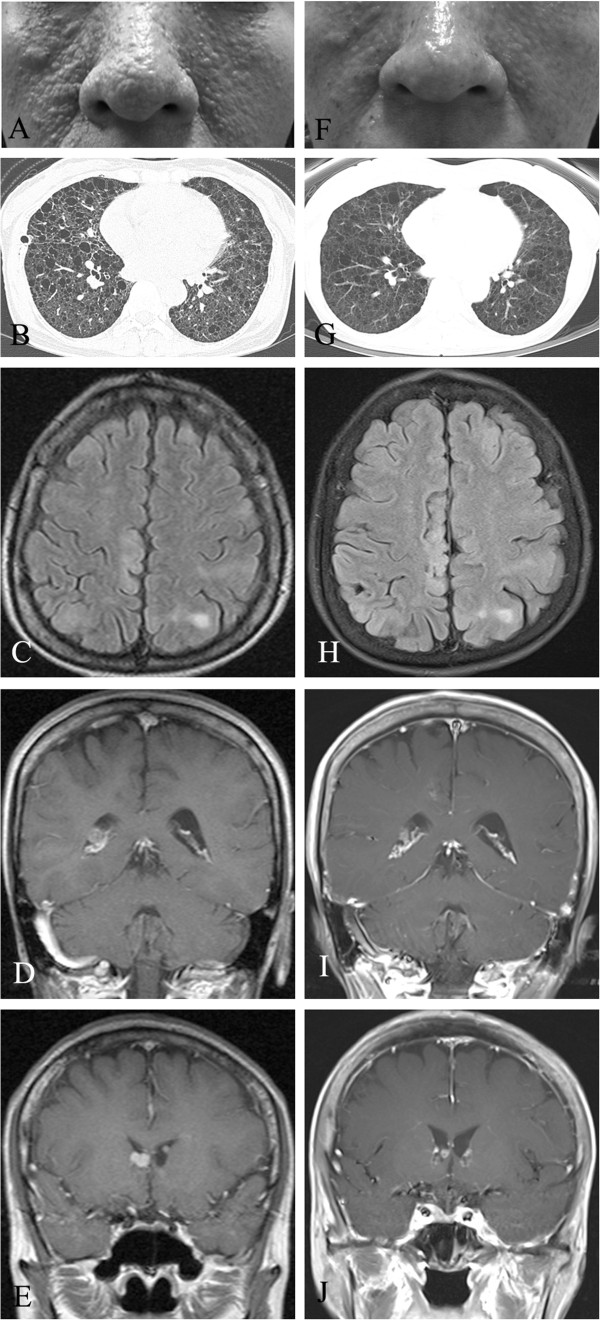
Clinical features of tuberous sclerosis complex at diagnosis and after treatment with everolimus. (A) Photograph of the patient’s facial fibroadenoma. (B) Chest computed tomographic scan of multiple cystic lesions consistent with lymphangioleiomyomatosis. (C) through (J) Brain magnetic resonance imaging scans of the patient. (C) Cortical tubers. (D) Multiple subependymal nodules. (E) Subependymal giant cell astrocytoma. (F) through (J) Changes after treatment with everolimus.
